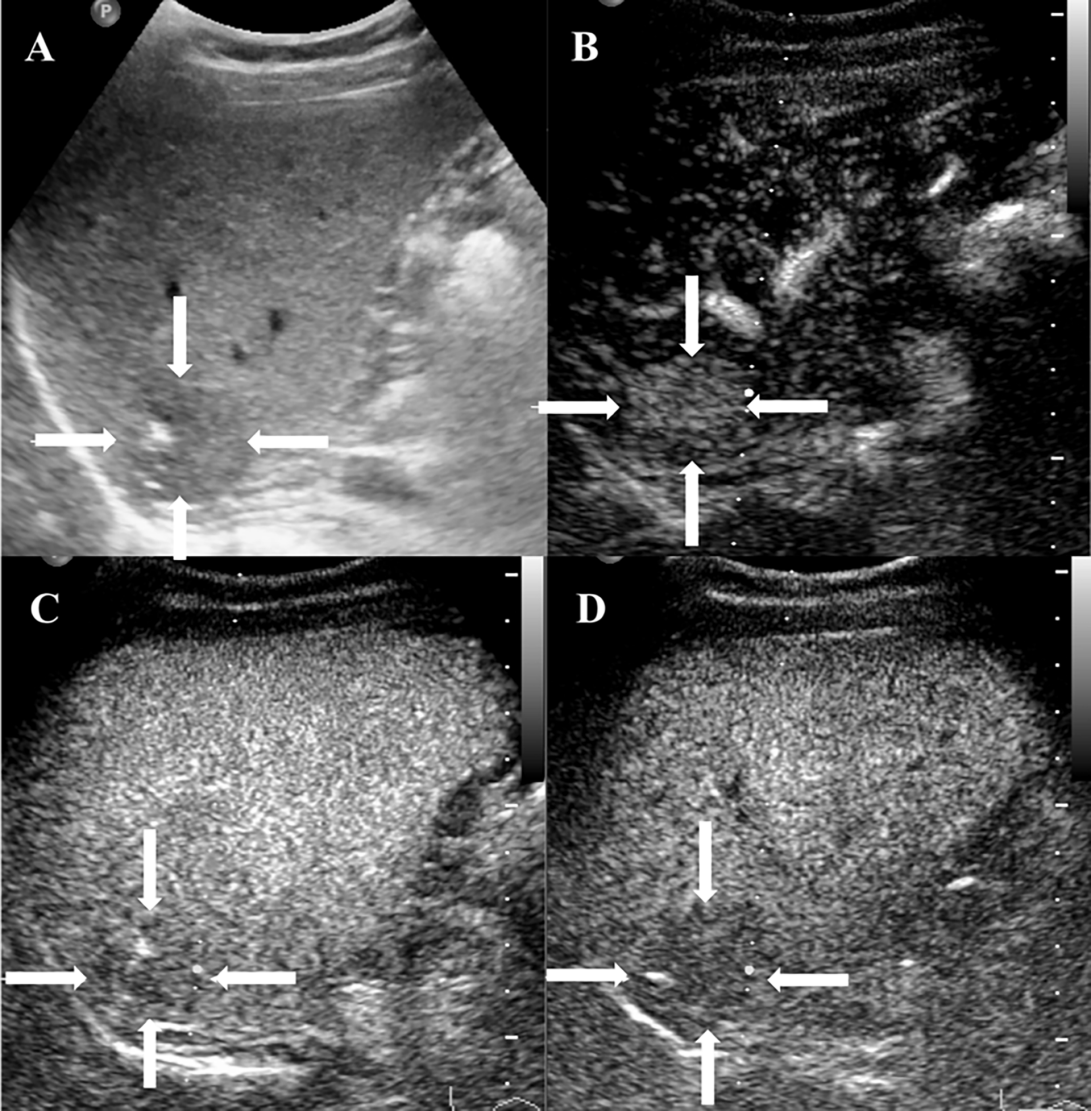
CEUS features of HEHE in a 38-year-old female. (A) Grayscale ultrasound displayed a subcapsular hypoechoic lesion (arrows) with ill-defined margin and calcification. (B) Homogeneous hyperenhancement (arrows) was seen in the arterial phase (12 s). (C) The lesion showed slight hypoenhancement (arrows) in the portal phase (85 s). (D) In the late phase (185 s), the lesion presented hypoenhancement (arrows).
